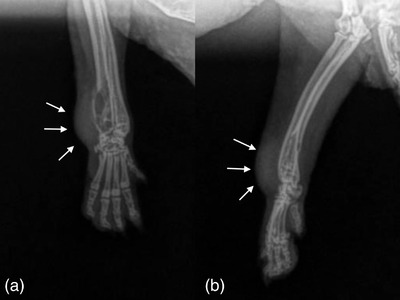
Dorsopalmar (a) and mediolateral (b) radiographs of the right antebrachium and manus of the hedgehog. There is a lytic, expansile lesion with an adjacent dorsolateral soft tissue mass effect overlying the right distal antebrachium
